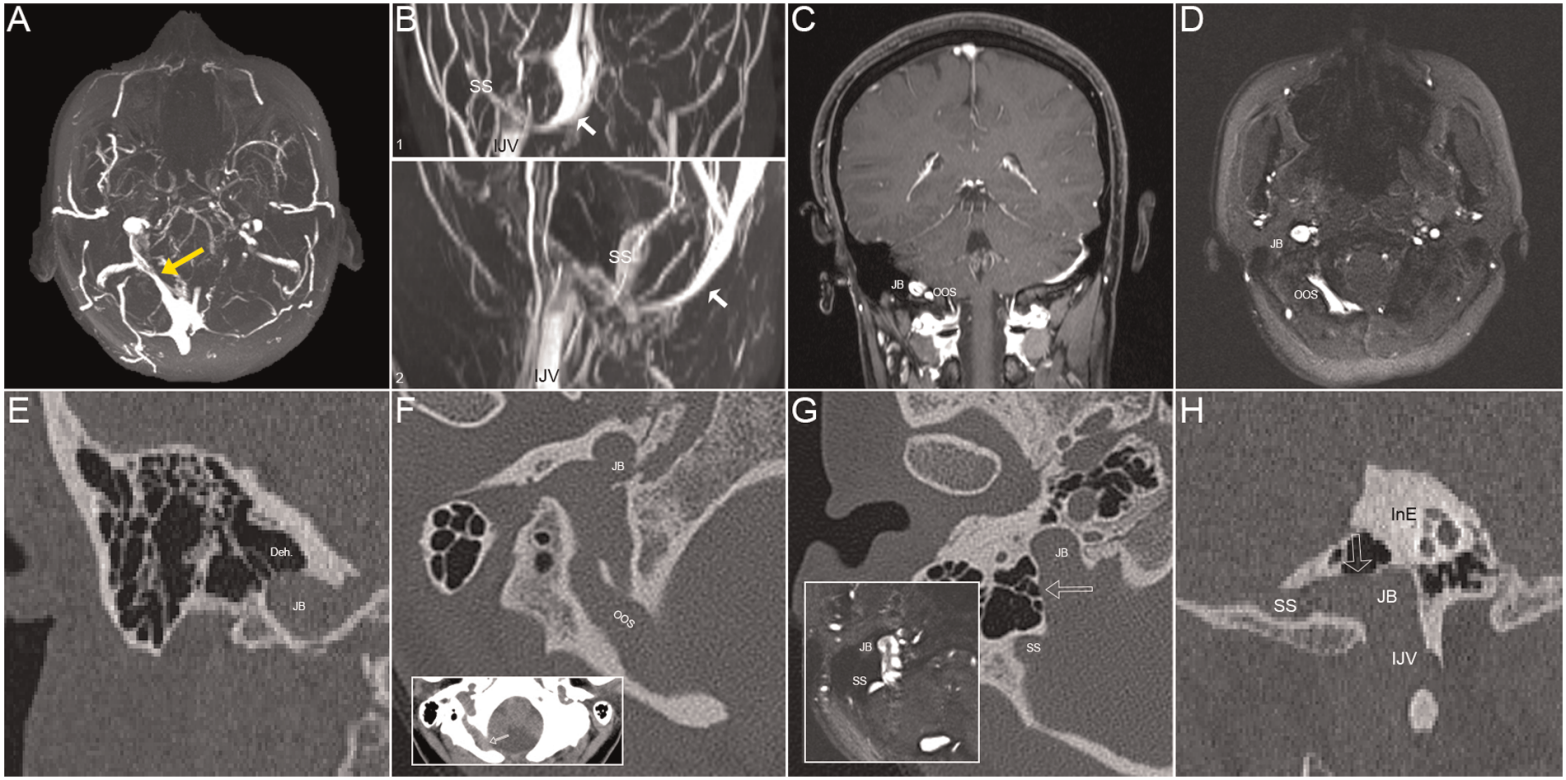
Radiological images of the patient in case 2 of right-sided pulsatile tinnitus (PT). (A) The maximum intensity projection (MIP) reconstruction of axial 2D-time-of-flight (TOF) magnetic resonance (MR) images showing an enlarged oblique occipital sinus (OOS) with distal stenosis (yellow arrow) anastomosing with the ipsilateral jugular bulb (JB). (B) (1) Coronal and (2) sagittal maximum intensity projection (MIP) reconstruction of 2D-time-of-flight (TOF) magnetic resonance (MR) images demonstrating the enlarged OOS (white arrows) running under the cerebellum. SS, sigmoid sinus; IJV, internal jugular vein. (C) Coronal contrast-enhanced T1-weighted gradient echo (2D FLASH) MR slice showing the relative locations of OOS and JB. (D) An axial 2D-TOF MR slice showing the SS, diverticulum (Div.), JB, and OOS. (E) Coronal CT image demonstrating dehiscence at the top of the SS-JB wall. (F) The notch of the OOS cuts through the edge of the foramen magnum of the occipital bone (red arrow). (G) Axial CT image showing SS-JB wall dehiscence and intrasinus flow pattern exhibited using the corresponding 2D-time-of-flight (TOF) magnetic resonance slice. (H) Reconstructed sagittal CT image exhibiting SS-JB wall dehiscence and locations of the SS, JB, and inner ear (InE).
